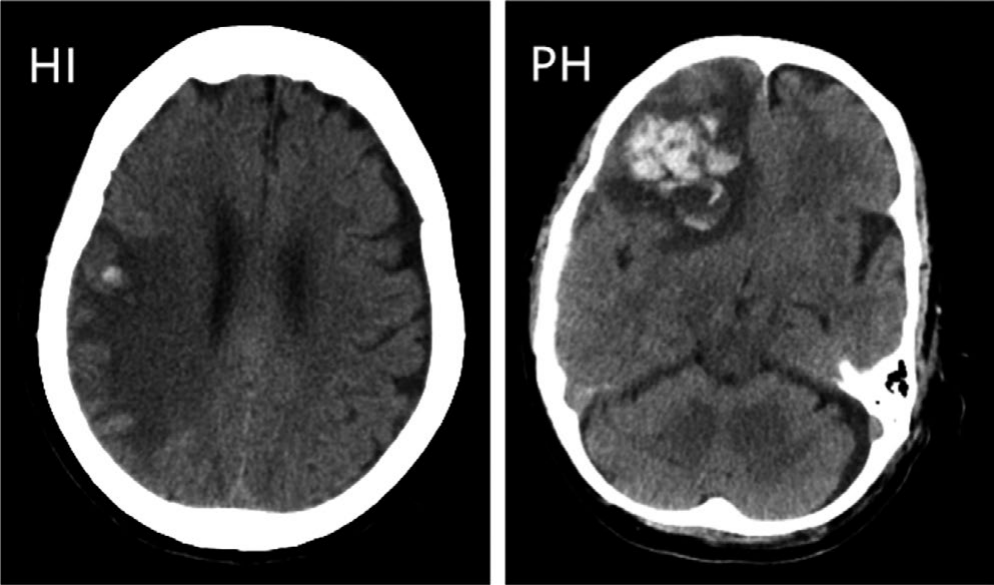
Hemorrhagic infarction and parenchymal hemorrhage. CT scan examples of hemorrhagic infarction (HI) and parenchymal hemorrhage (PH), according to the European Cooperative Acute Stroke Study II classification
